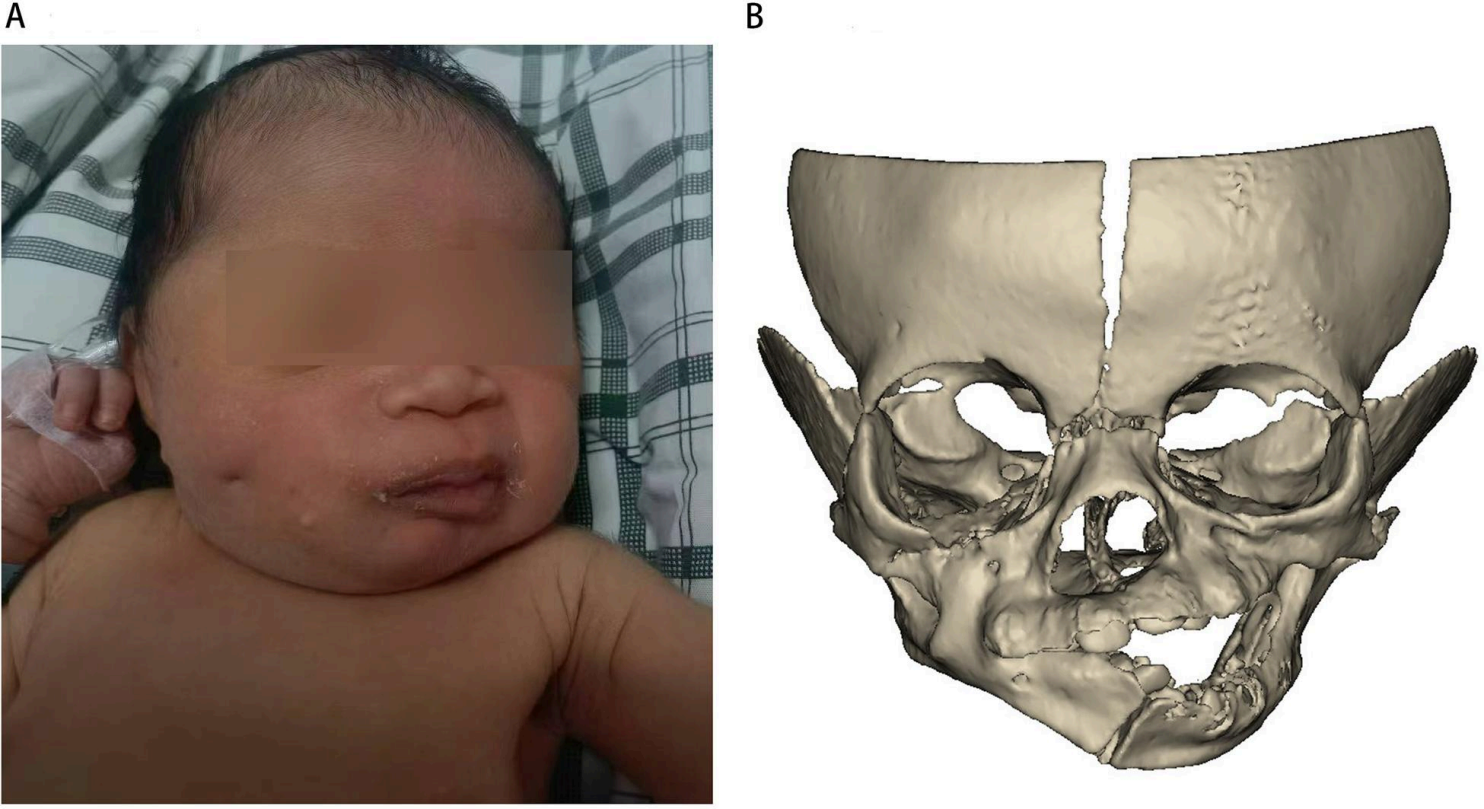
(A) Frontal photograph of the neonate showing limited mouth opening and facial jaundice. (B) Frontal 3D CT reconstruction demonstrating unilateral bony fusion on the right side.
